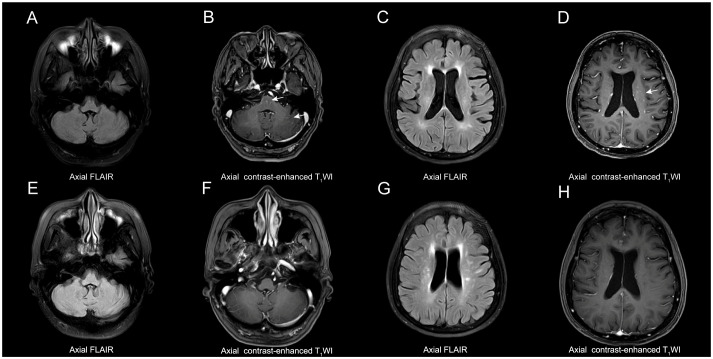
Brain MRI images at first relapse (A–D) and during remission (E–H). (A–D) Multiple areas of mild FLAIR hyperintensity in the bilateral cerebral hemispheres, brainstem, and cerebellum, with enhancement. (E–H) Reduced extent or disappearance of multiple enhancing lesions in the cerebral hemispheres, brainstem, and cerebellum. MRI, magnetic resonance imaging; T_1_WI, T_1_-weighted imaging; FLAIR, fluid-attenuated inversion recovery.
